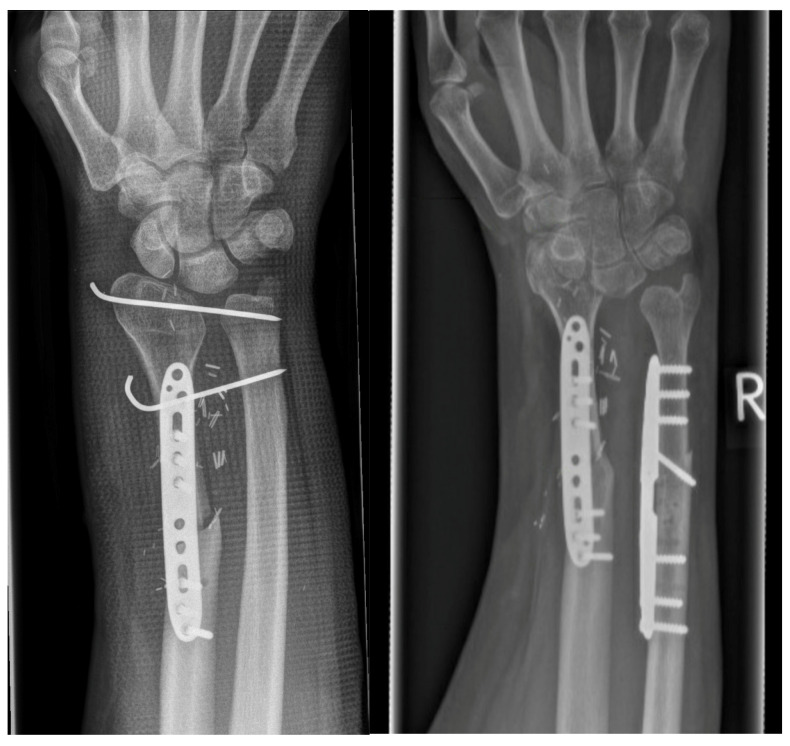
First postoperative X-ray. The fibular head graft and distal ulna were transfixed (left). One year later, the ulna was shortened, while wrist function remained good despite the narrowing of the new proximal wrist joint with subluxation (right).
